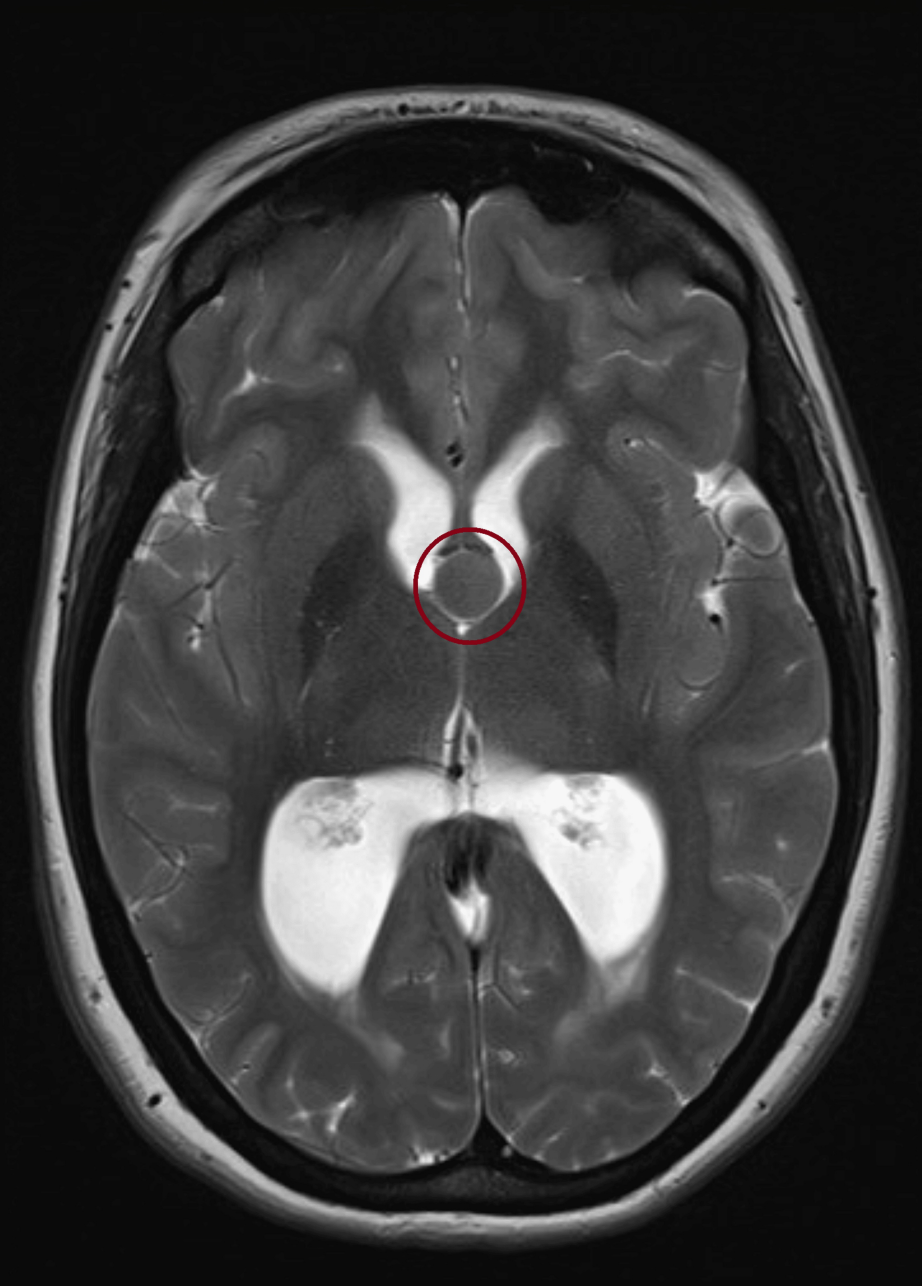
Axial T1-weighted MRI demonstrating a hyperintense, well-defined colloid cyst at the foramen of Monro with associated ventricular dilation.
